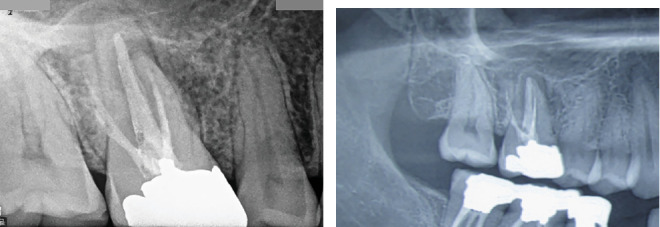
Radiographic evaluation revealed a substantial periapical radiolucency encircling the apices of the MB, DB, and P roots. (a) Periapical view and (b) a part of the panoramic view.
